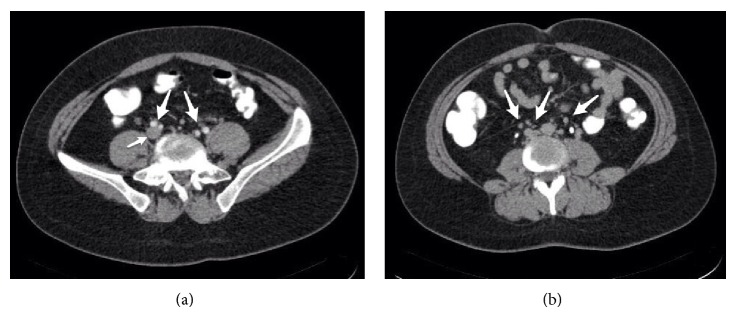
Axial CT image shows the patent left and right internal and external iliac arteries (arrows) and the patent right common iliac vein (small arrow) (a). Axial CT image from a lower level shows the collateral vascular structures (arrows) (b).
